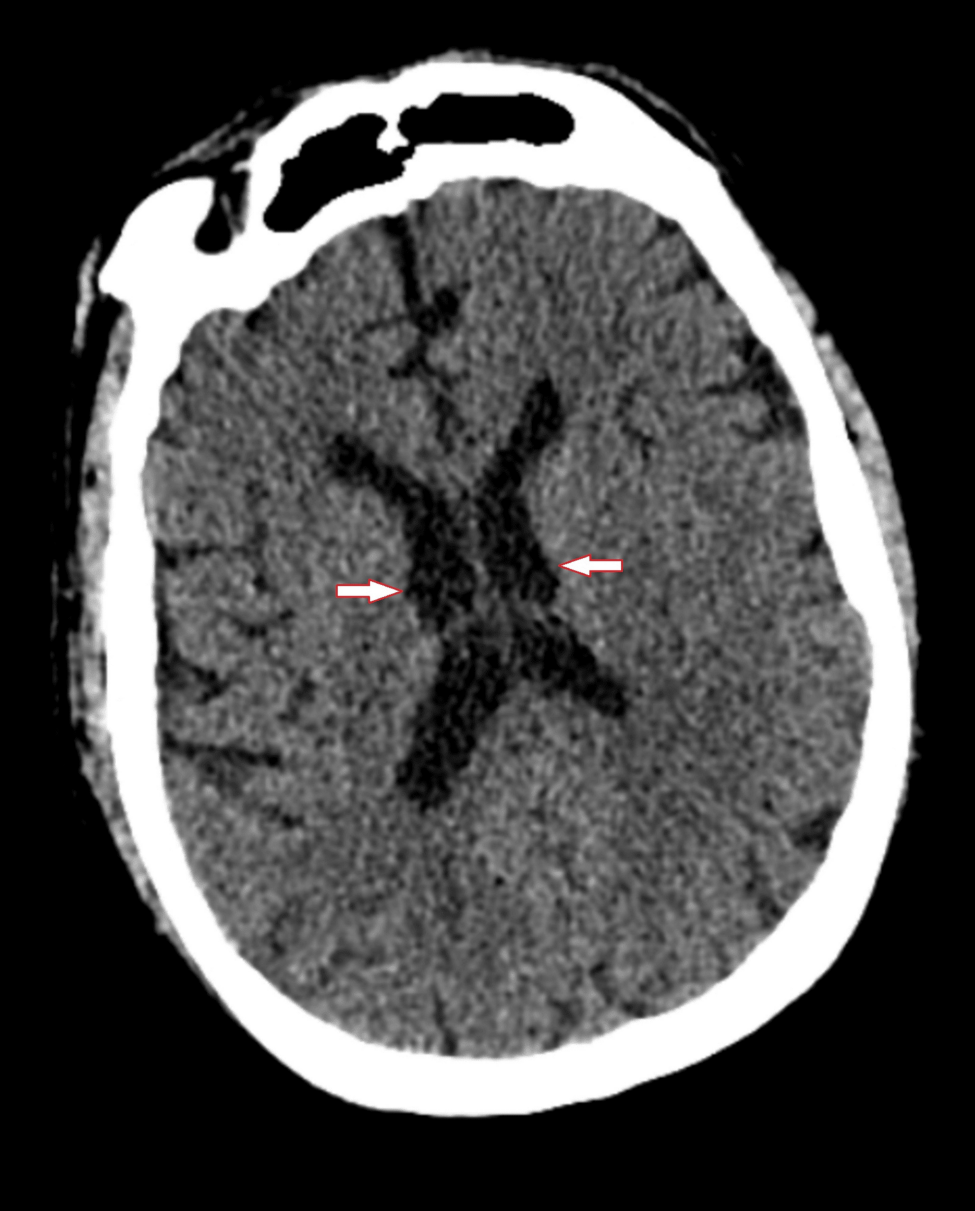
CT of brain without contrast showing lateral ventricles are normal in size and configuration. No hydrocephalus, midline shift, or extra-axial fluid collection.
